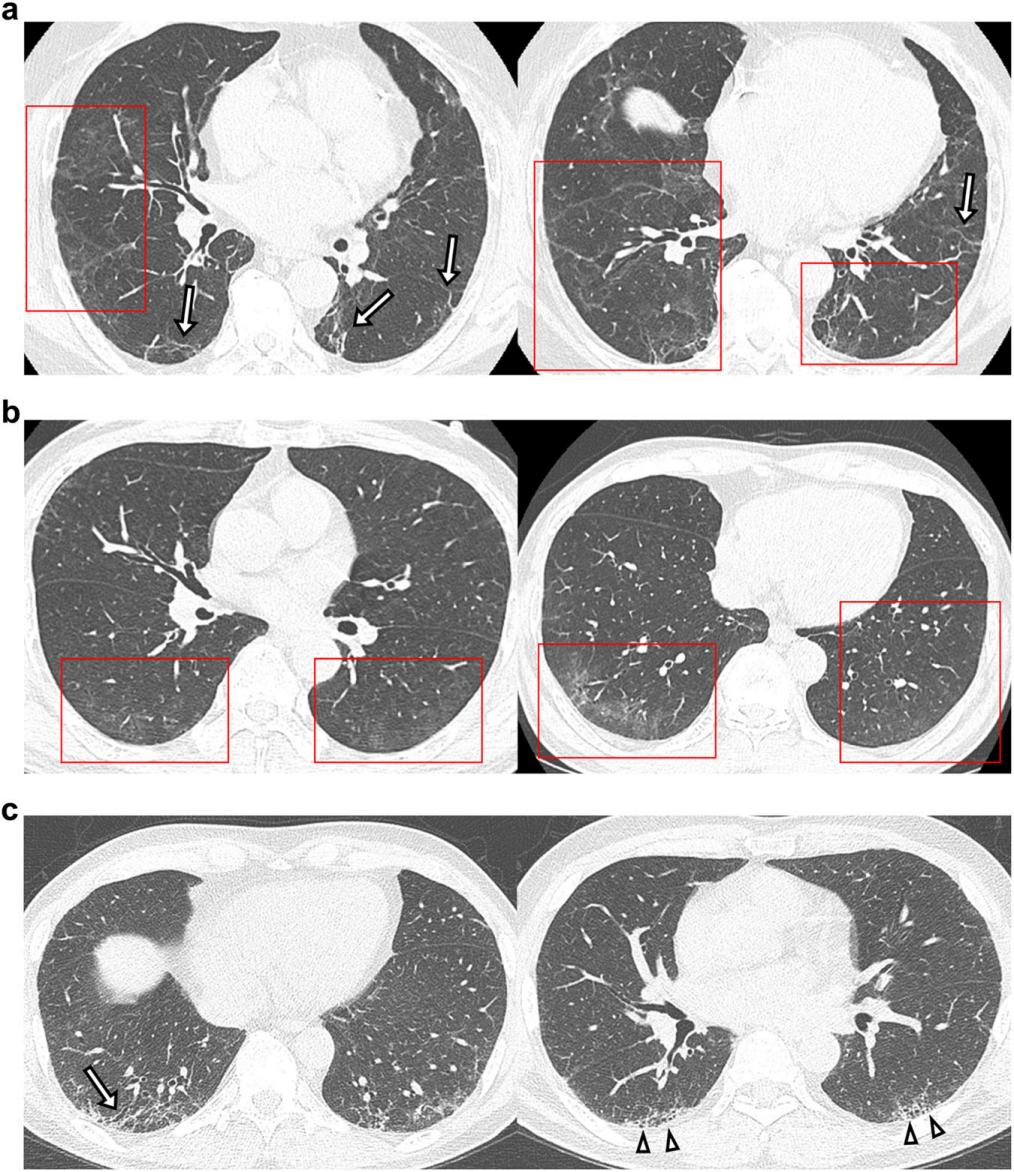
a Representative case of post-COVID-19 residual abnormalities. Unenhanced HRCT images show extensive GGO as the predominant finding, with a peribronchovascular distribution. Perilobular opacities (red squares) and parenchymal bands (arrows) are also present. b Representative case of non-fibrotic ILA. HRCT images show GGO as the predominant finding, with a subpleural and basal distribution (red squares). c Representative case of fibrotic ILA. HRCT images show reticulation as the predominant finding, with a subpleural and basal distribution. Traction bronchiolectasis (arrow) and non-emphysematous cysts (arrowheads) are also present
